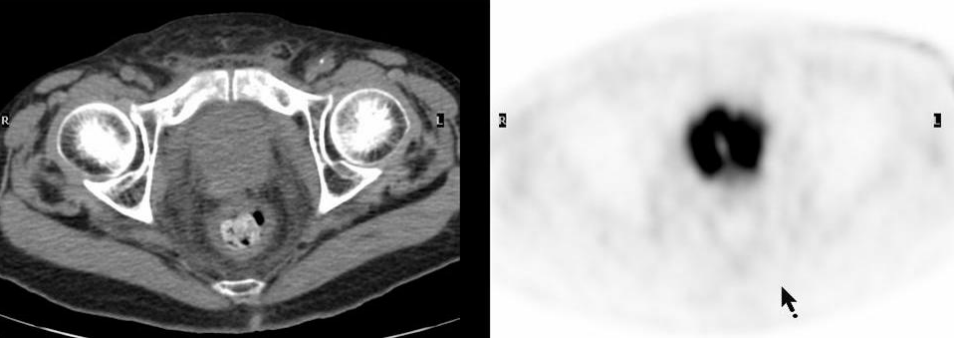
Neuroendocrine differentiation of the prostate cancer displays high grade of FDG uptake on transaxial PET-CT images, which is different from that noted in most of adenocarcinoma of the prostate.
